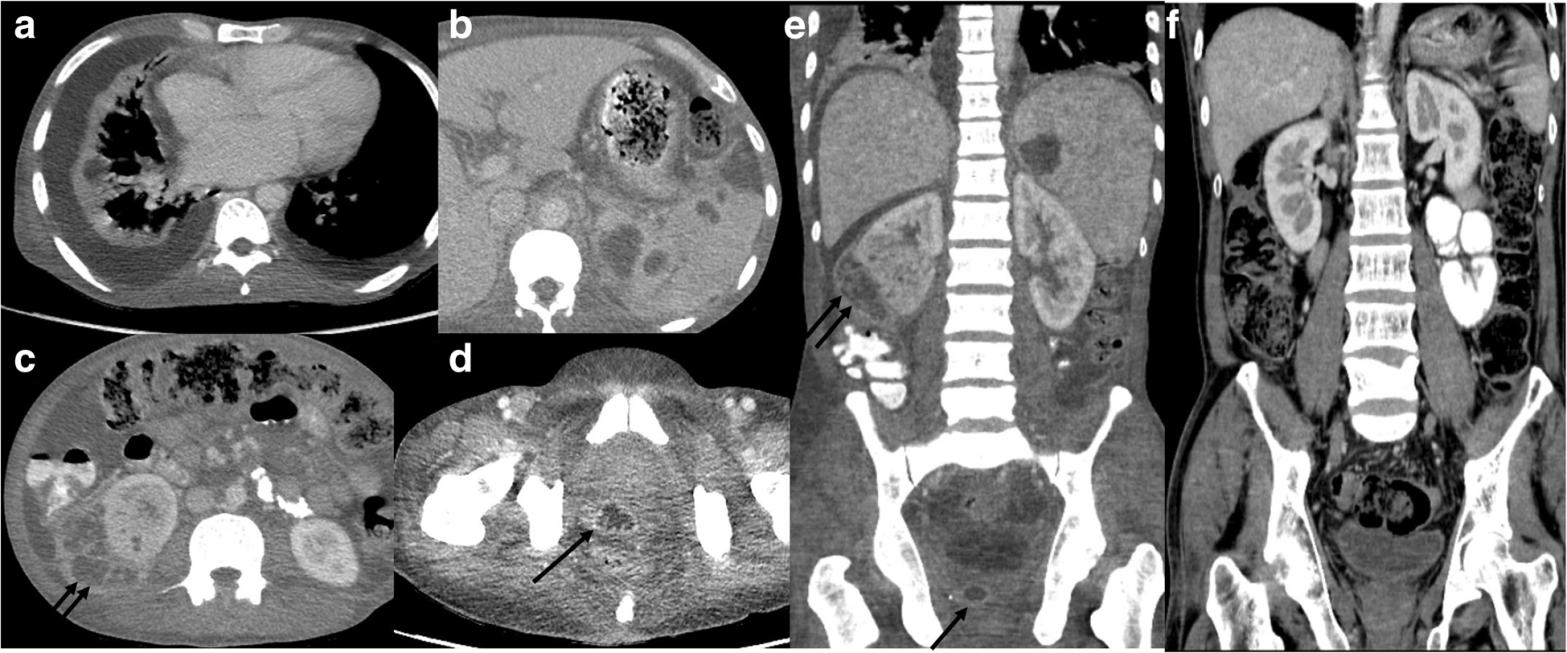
Disseminated melioidosis. Forty-three-year-old gentleman with pyrexia of unknown origin and anaemia, axial CT lower thorax reveal (a) bilateral pleural effusion with small evolving abscess in the atelectatic lung, bilateral pleural effusion, (b) multiple splenic abscesses, (c) large right renal abscesses with large subcapsular collection (double arrow), (d) prostatic abscess (single arrow). e Coronal CT abdomen images also demonstrate the same findings. f Follow-up CT of the abdomen after 6 months revealed complete resolution of the abscesses in various organs
